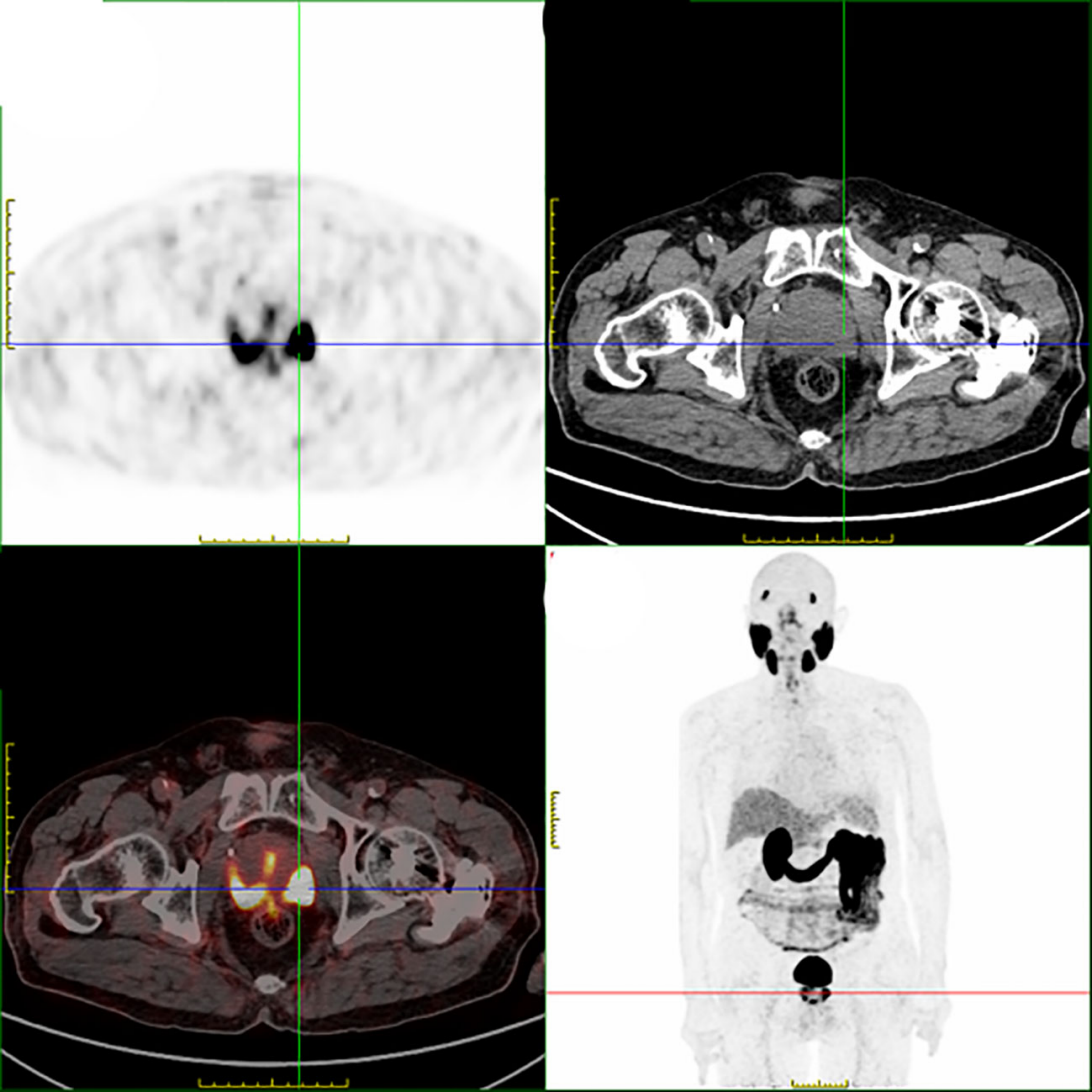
Enlarged prostate with multiple foci of high PSMA expression in the prostate, considering malignant lesions.
